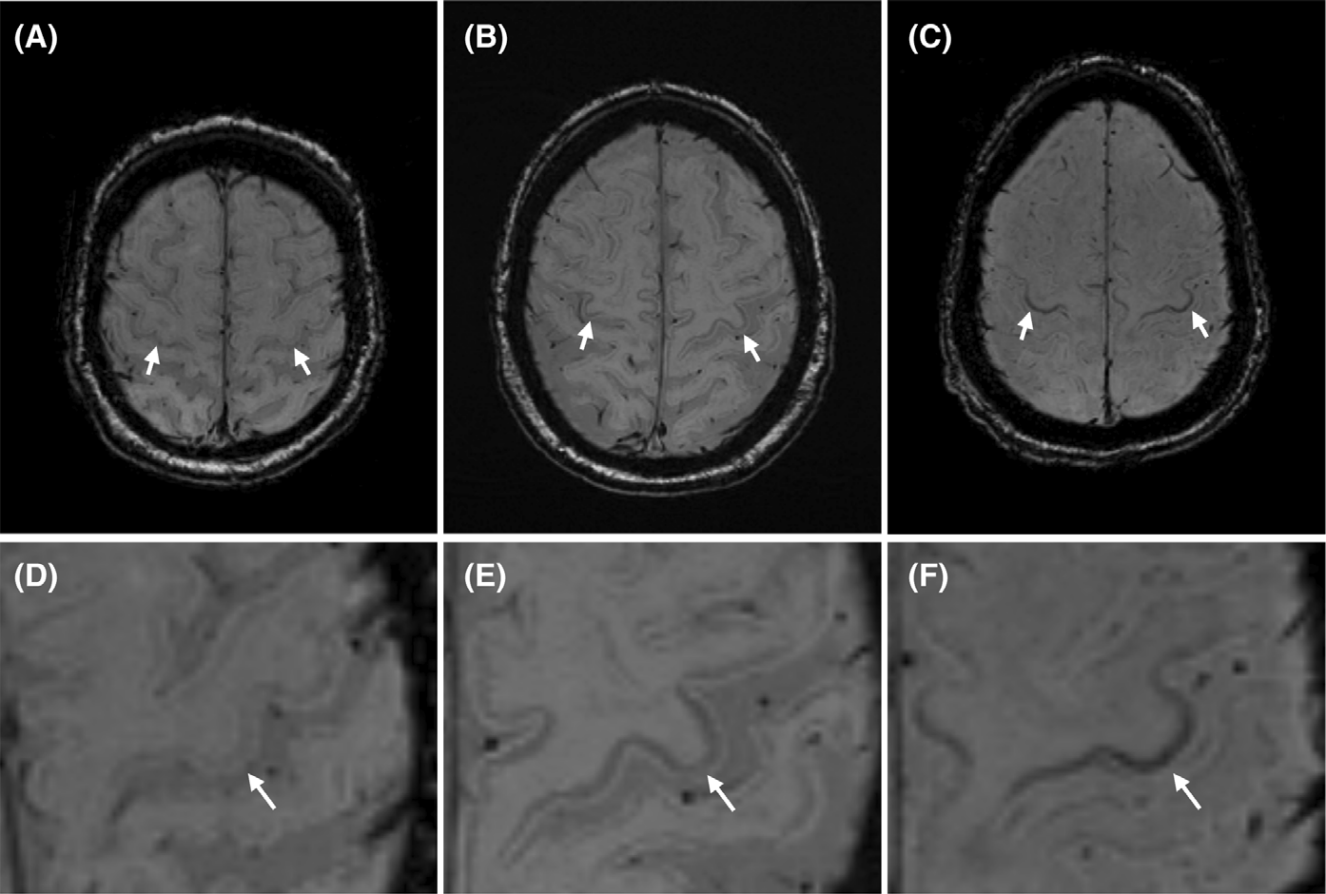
Motor band sign grading. Different grades (0–2) of primary motor cortex hypointensities, the motor band sign, on axial susceptibility‐weighted brain MRI in the intermediate region. D–F showing magnifications of A–C, respectively. A 74‐year‐old male with isointense (Grade 0) motor cortex (D); a 73‐year‐old male with mildly hypointense (Grade 1) motor cortex (B and E), and a 70‐year‐old male with marked hypointense (Grade 2) motor cortex (C and F).
